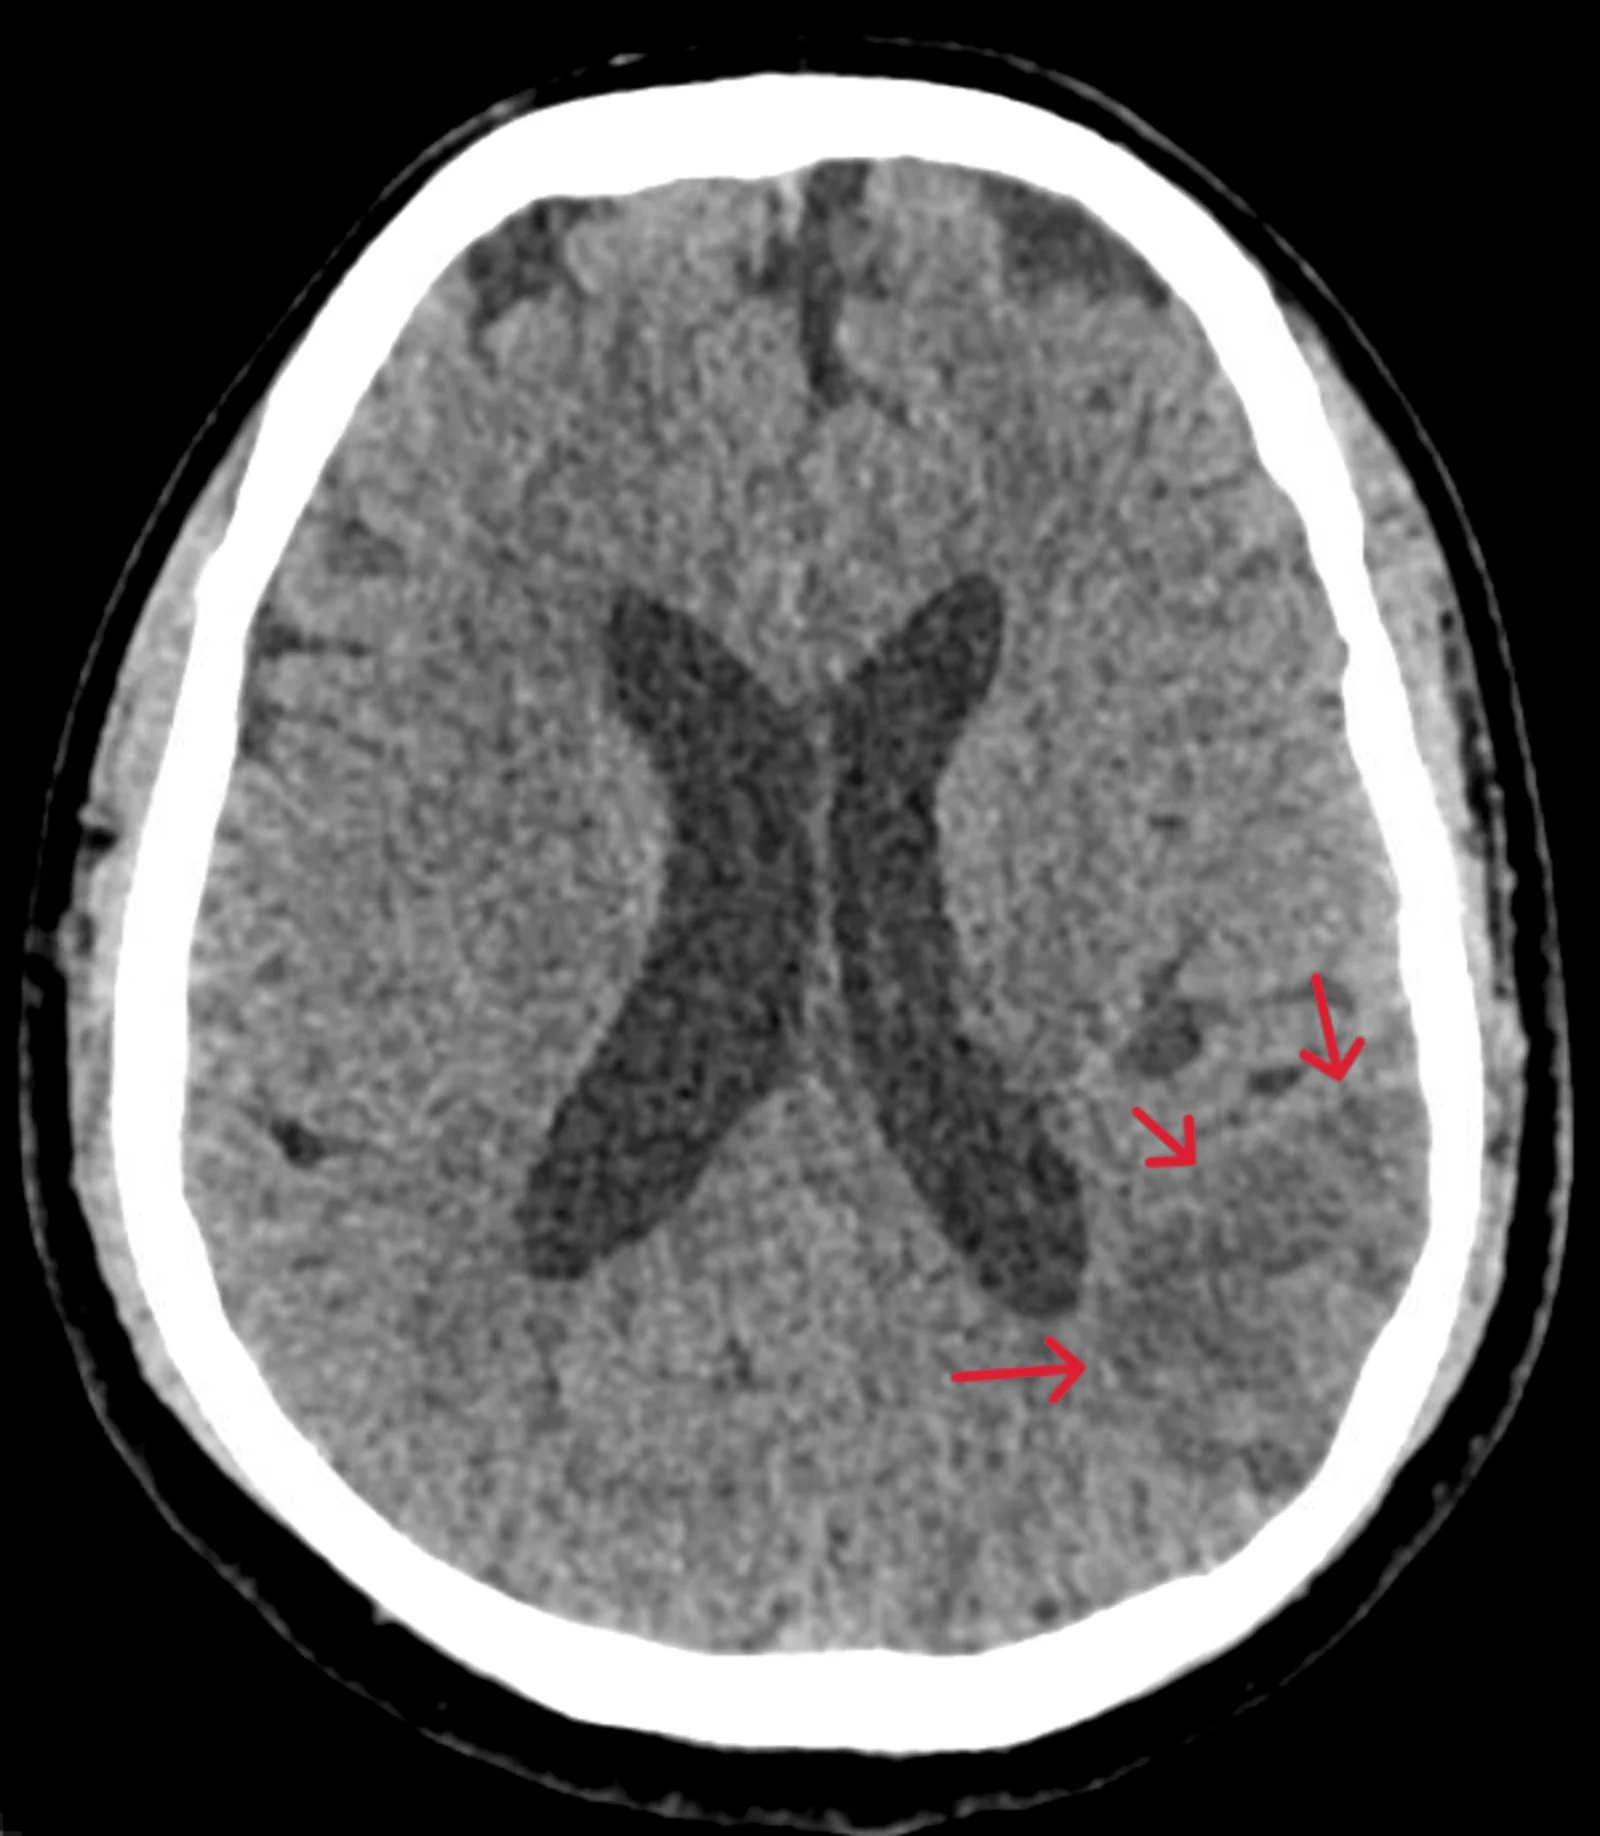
Non-contrast computed tomography (CT) brain showing established hypodensity in the left parieto-occipital region, consistent with acute infarction (as indicated by the red arrows).
